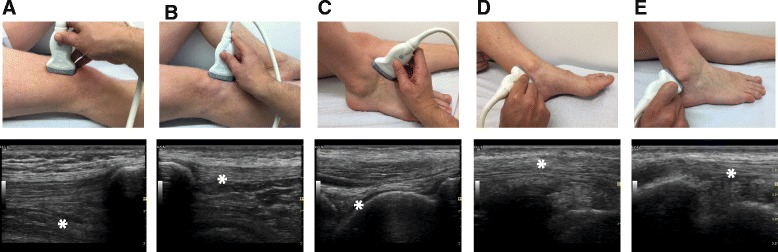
Overview of the acquired aspects with their normal ultrasound image. a suprapatellar longitudinal axis (* suprapatellar recessus); b infrapatellar longitudinal axis(* proximal patellar tendon enthesis); c dorsal longitudinal axis of the talocrural joint (* joint margins of the upper talocrural joint); d longitudinal axis of the medial ankle tendons (* posterior tibial tendon); e longitudinal axis of the lateral ankle tendons (* long and short peroneal tendons)
